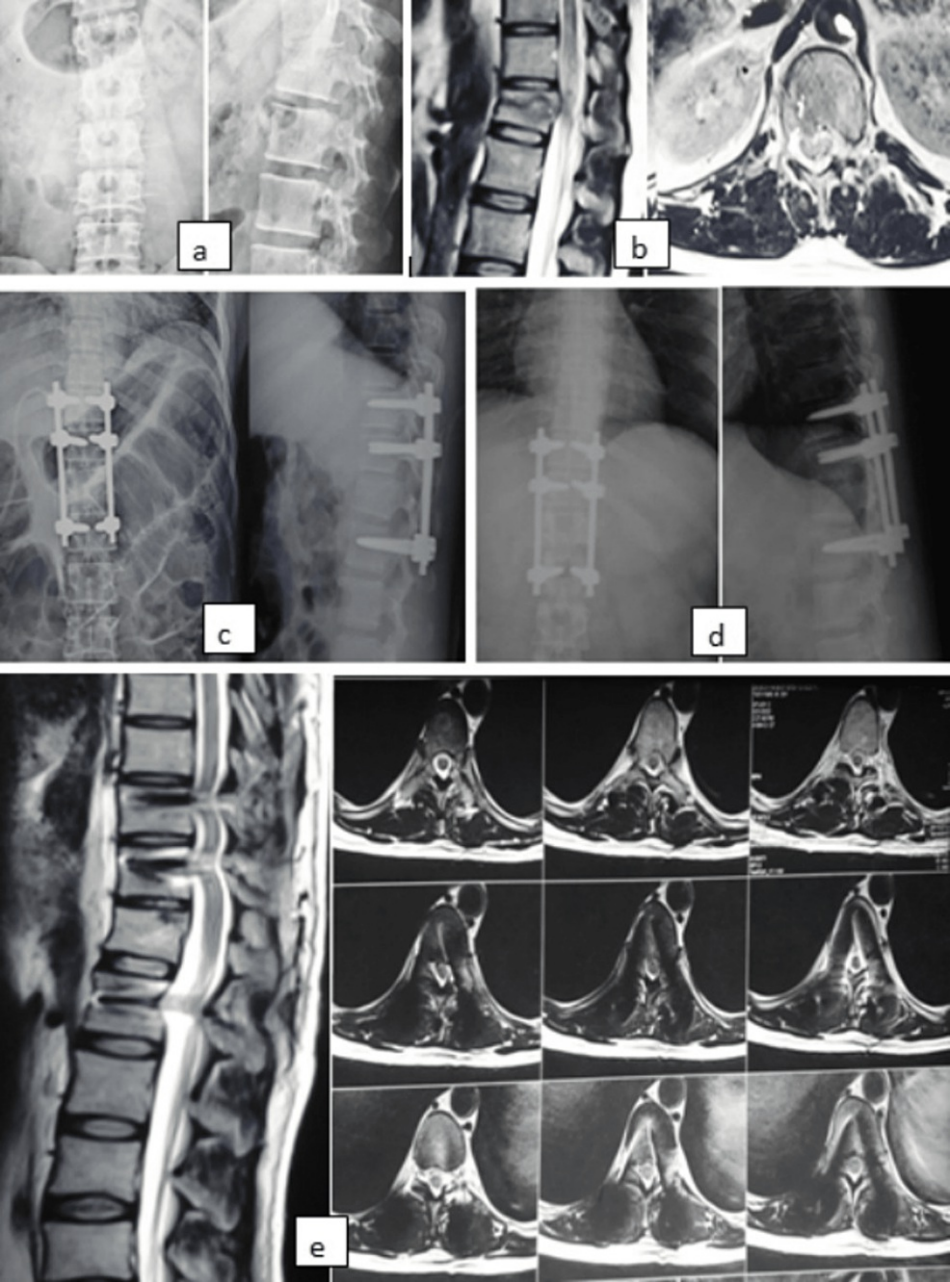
Case example showing (a) preoperative x-ray AP and lateral view showing D12 vertebra fracture with kyphosis angle of 18ᵒ. (b) Preoperative MRI showing burst fracture of D12 vertebra with cord edema and spinal canal occlusion of 20% and 30% in sagittal and axial view, respectively. (c) Immediate post-operative radiographs (kyphosis - 4ᵒ) and (d) final follow-up radiographs at 12 months (kyphosis - 8ᵒ) showing correction of kyphosis and maintenance. (e) MRI at 12 months follow-up showing restoration of vertebral height and resolution of cord edema and spinal canal occlusion.
